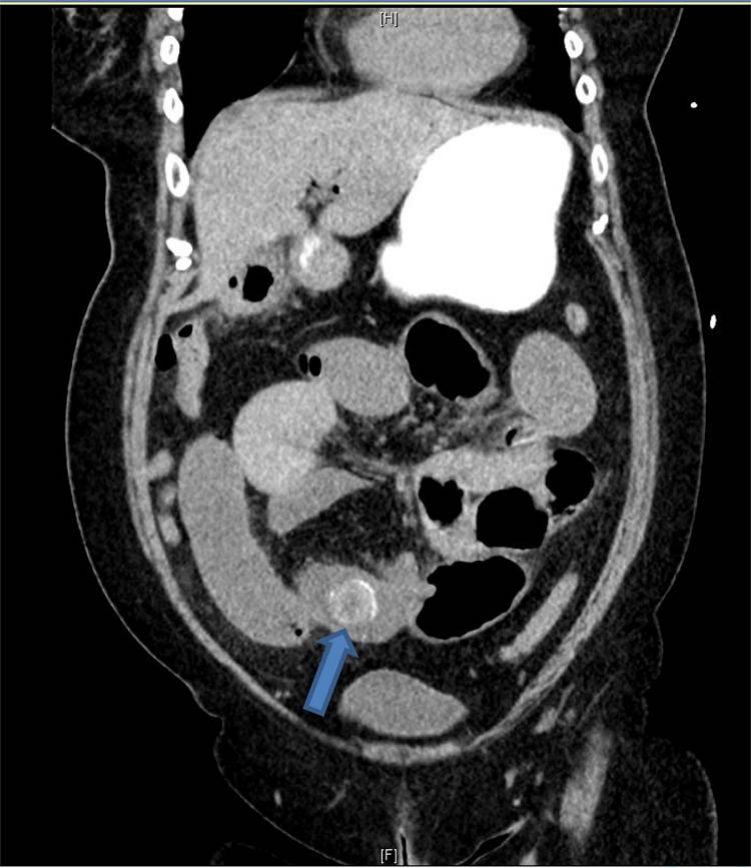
Coronal CT image demonstrates gallstones (blue arrow) (only one gallstone shown on this image) in a loop of small bowel in the right lower quadrant.
